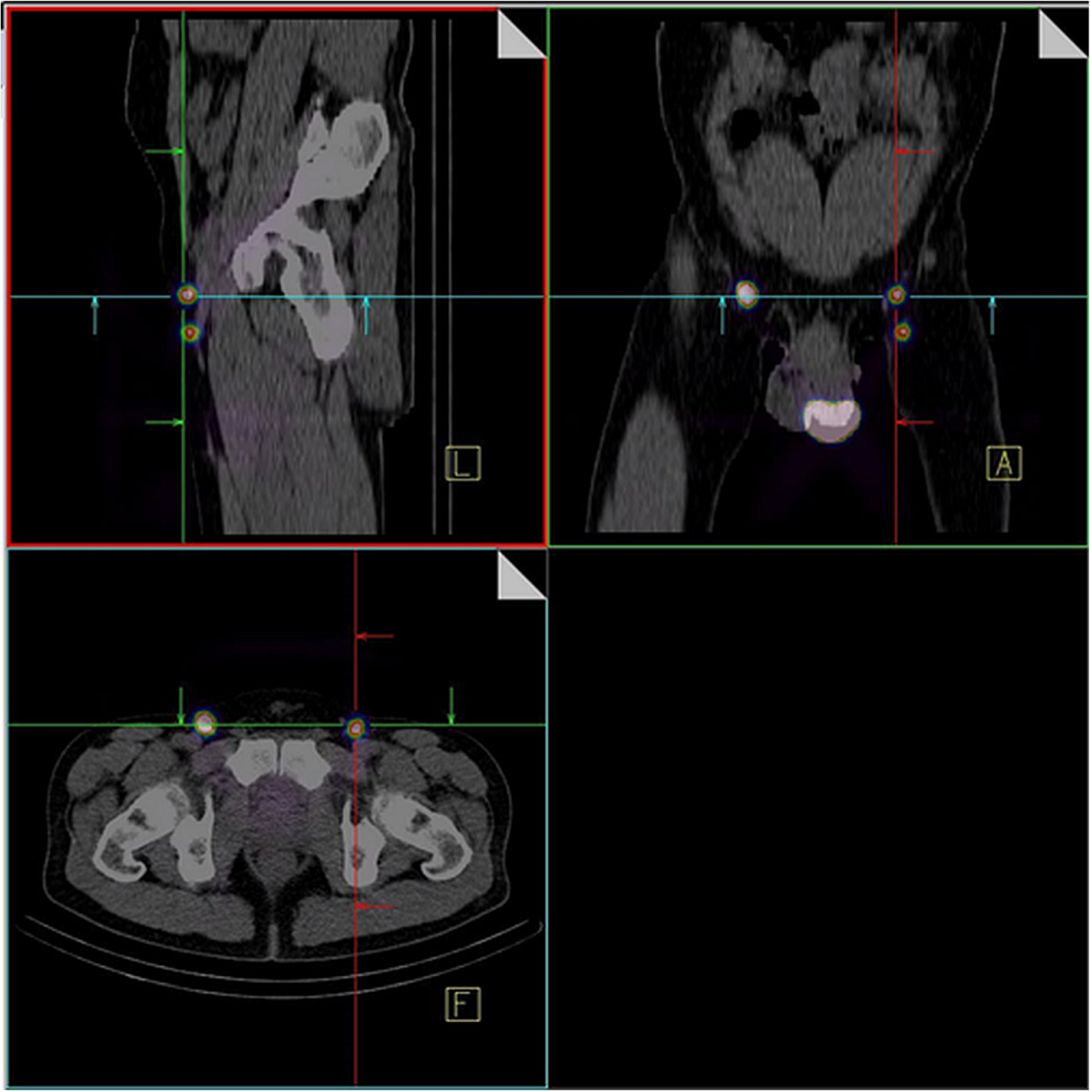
Pre-operative scans of sentinel lymph nodes by means of SPECT/CT: Evidence of so-called “hot spots” in the inguinal region bilaterally (2 SLN left, 1 SLN right) as well as in the region of the tumour
